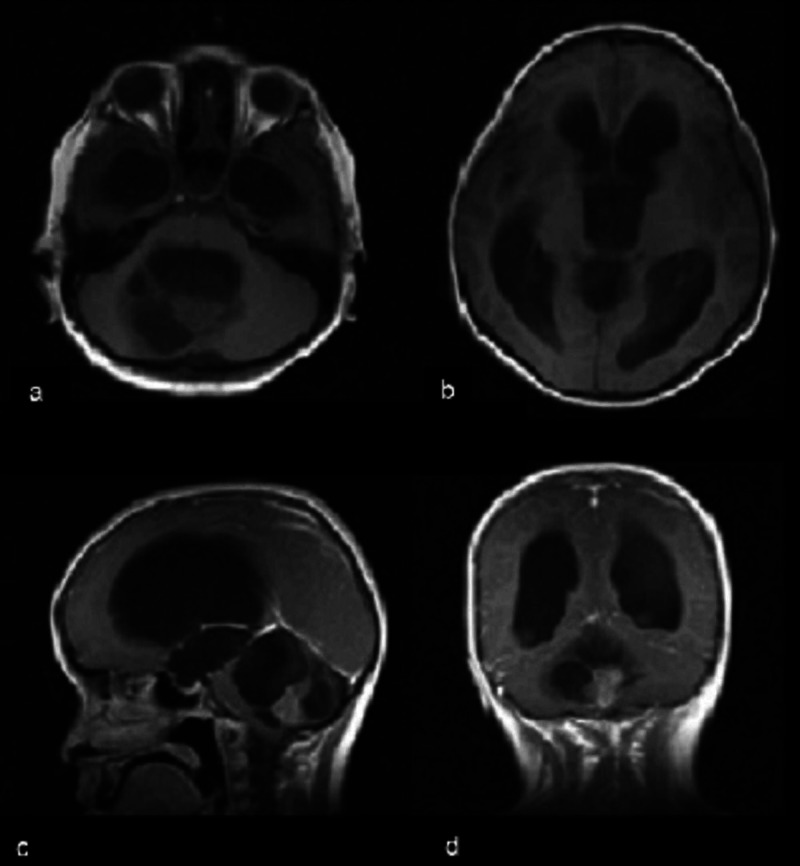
Pre-operative brain MRI A, B: axial non-contrast T1 weighted image demonstrating an isointense lesion protruding into the fourth ventricle causing obstructive hydrocephalus. C: Contrast-enhanced T1-weighted MRI, sagittal view. D: Contrast-enhanced T1-weighted MRI, coronal view.
